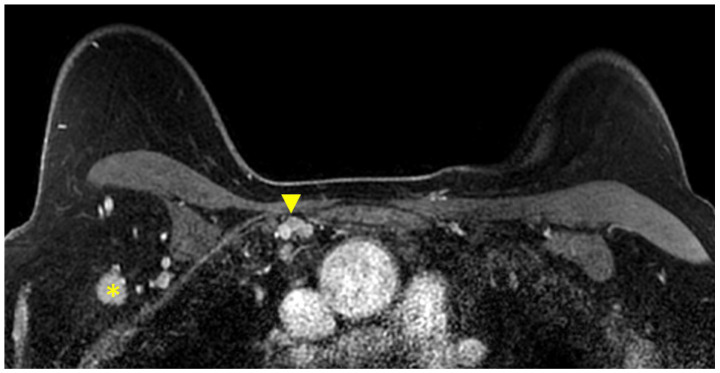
Axial T1W fat-suppressed post-contrast breast MRI provides a global view of the axillary and internal mammary nodal basins. In this case, level I axillary adenopathy (asterisk) and internal mammary adenopathy (arrowhead) are present.
